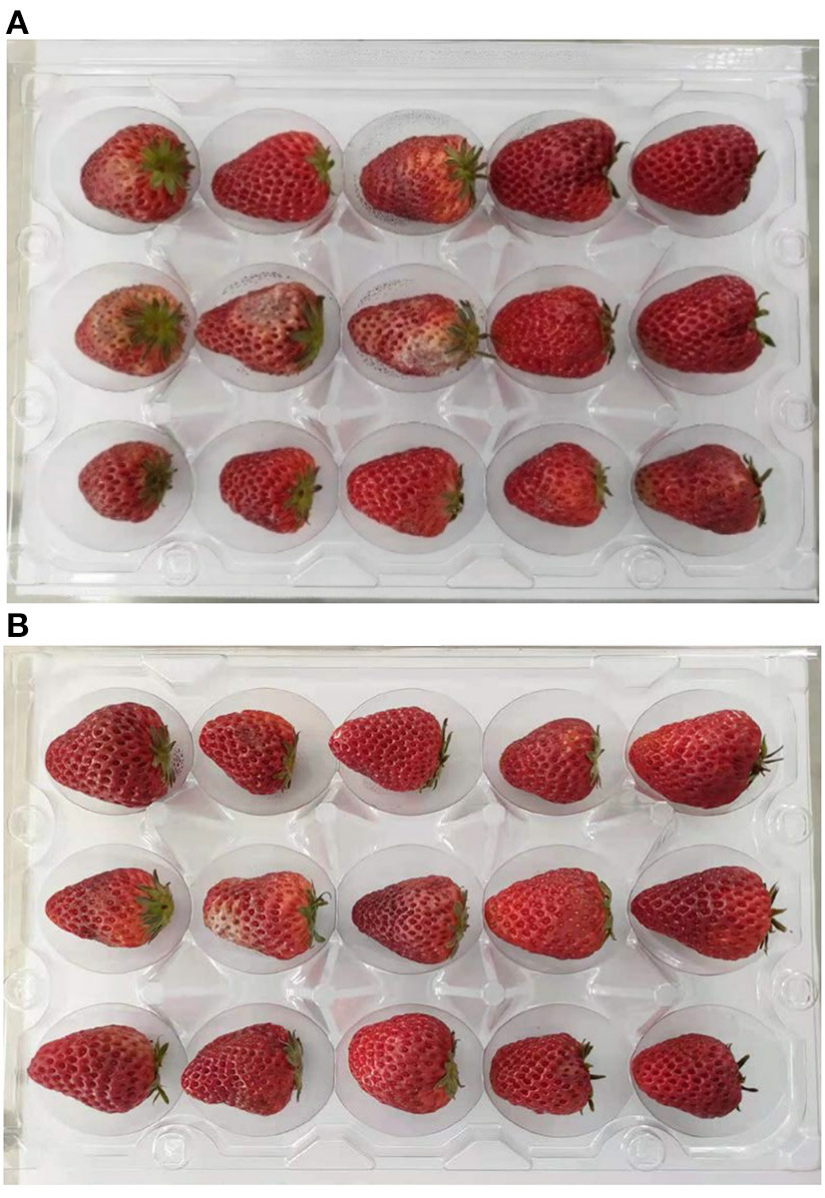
Appearance of the control (A) and ultrasound (B) groups at 24 d, ultrasound treatment condition was frequency 28 kHz, power 0.15 W/cm^2^, time 3 min.
